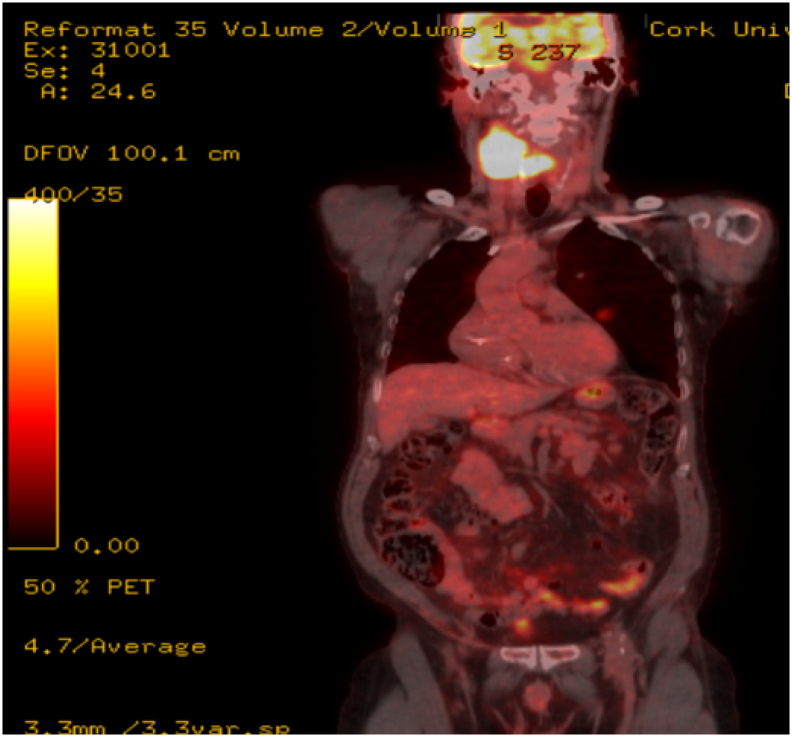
PET scan showing FDG avid uptake in the neck, liver deposits, and pulmonary nodules.
